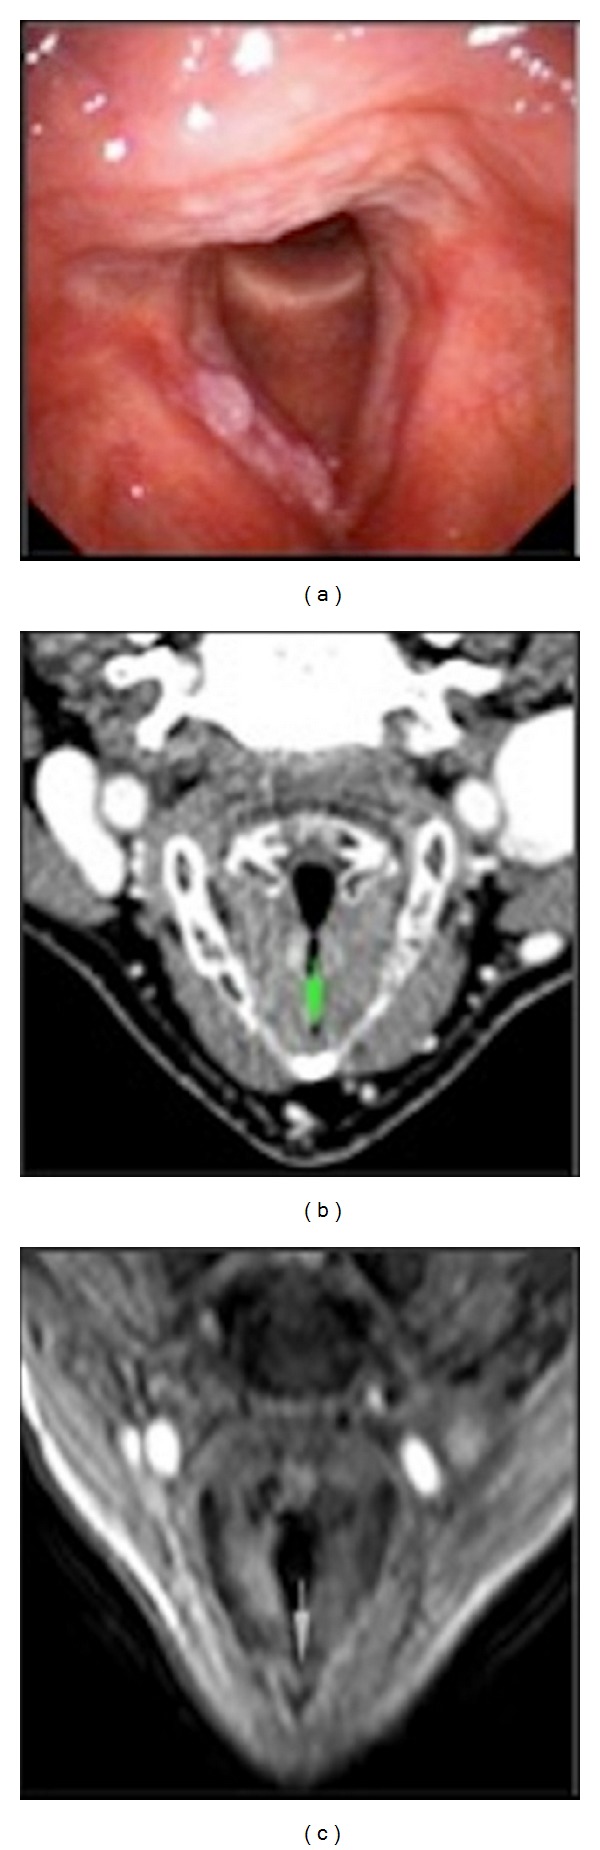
Bilateral glottic carcinoma with involvement of anterior commissure: (a) endoscopic view; (b) CT image: contrast enhancement of right vocal fold, but the commissure seems preserved (green indicator); (c) T1w MR image after contrast shows involvement of right true vocal cord, anterior commissure, and anterior part of left vocal fold (green indicator).
